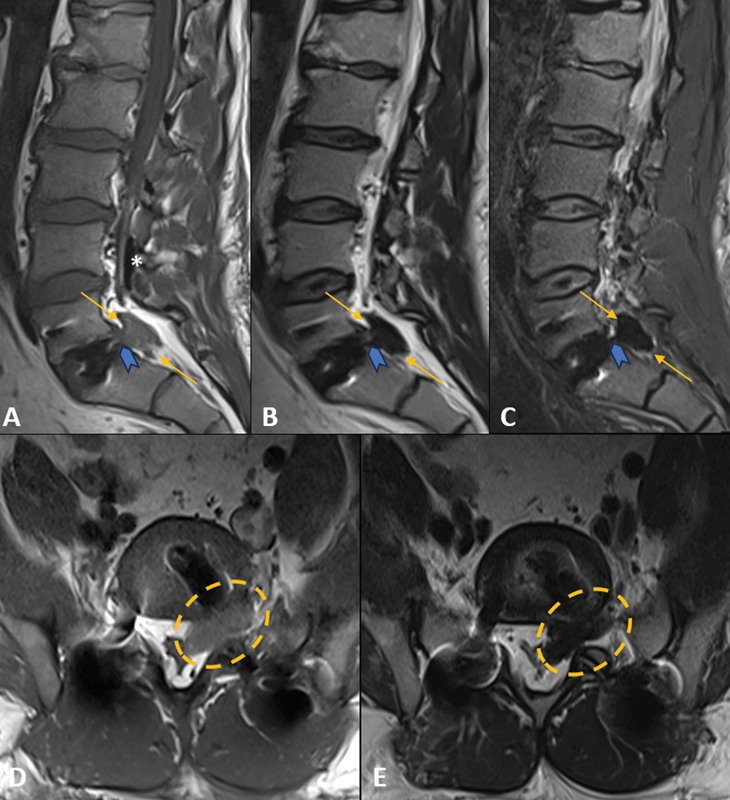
Postoperative lumbar spine magnetic resonance imaging after 1-day. (
